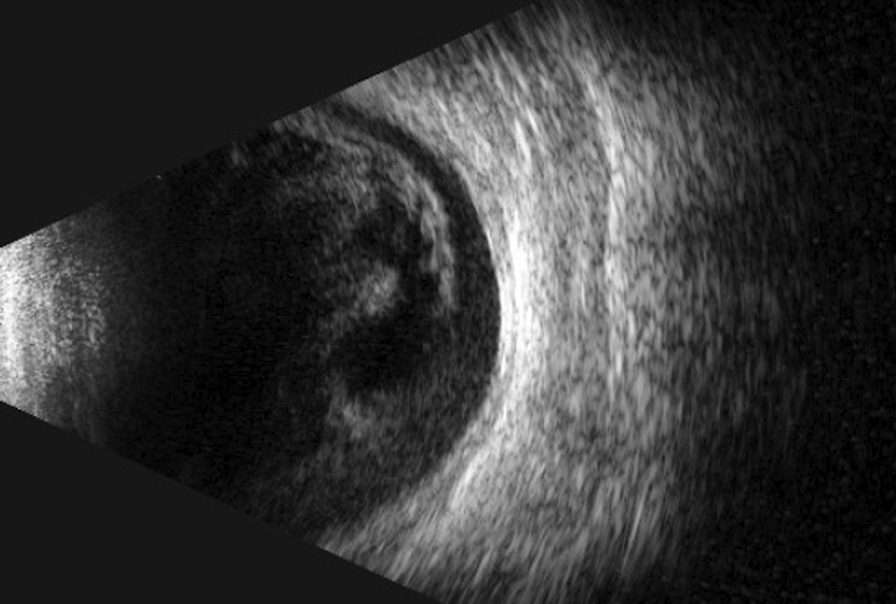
B-scan ultrasonography showed extensive vitreous opacities and an attached retina
